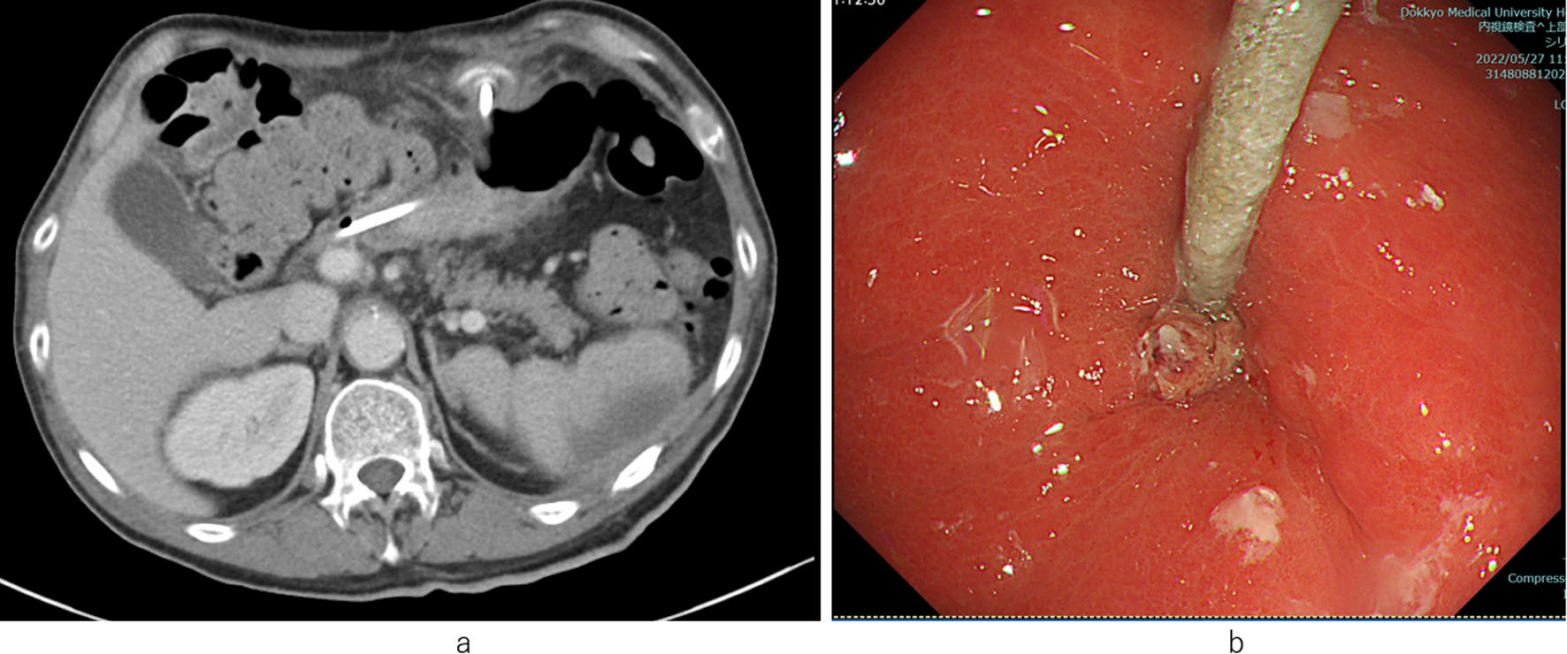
Preoperative imaging findings. a CT revealed an internal bumper of PEG-J buried in the gastric wall. b EGD showed a complete BBS of PEG-J. PEG-J, percutaneous endoscopic gastrostomy with a jejunal extension tube; BBS, buried bumper syndrome; *EGD* esophagogastroduodenoscopy; *CT* computed tomography
